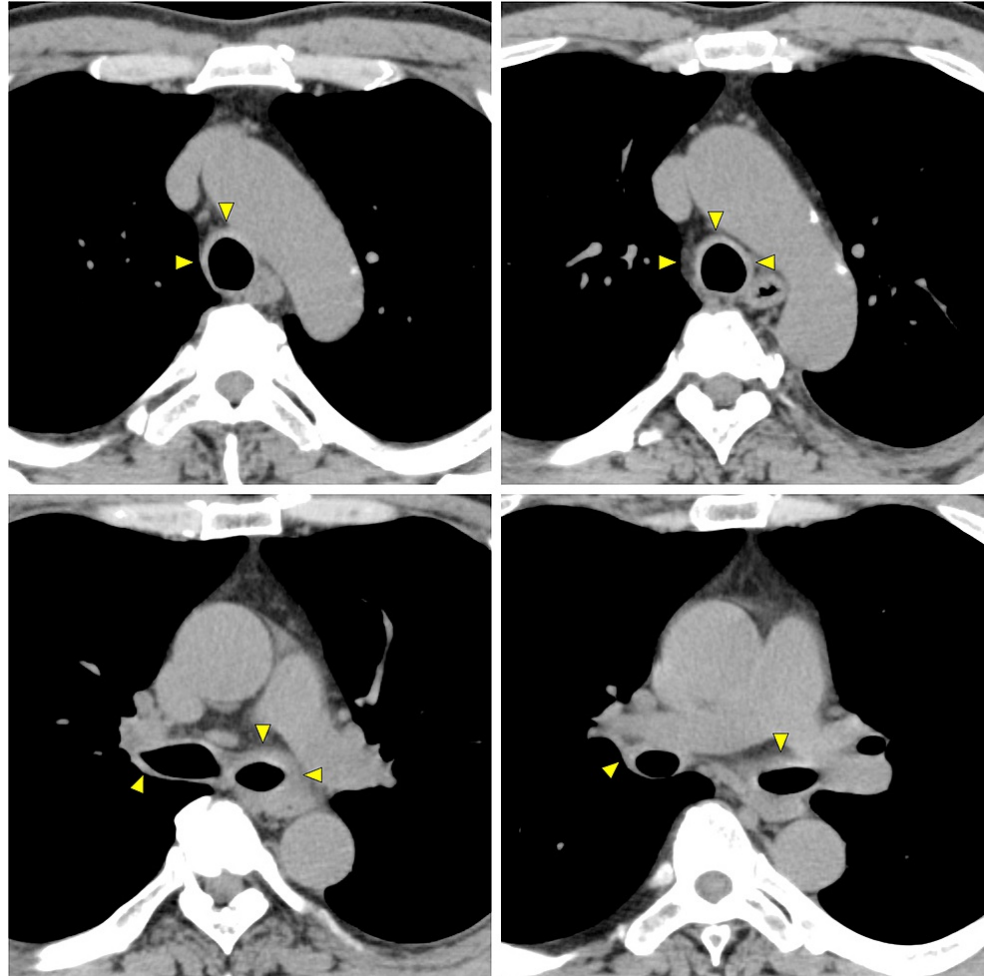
Chest CT images of the patient at the initial presentation. Thickened tracheal and bronchial walls (arrows) with no active lesions in the lung. There was no nodular wall thickening or gross calcification. There was a mild increase in the concentration of soft tissue around the trachea, with slightly enlarged mediastinal lymph nodes.
